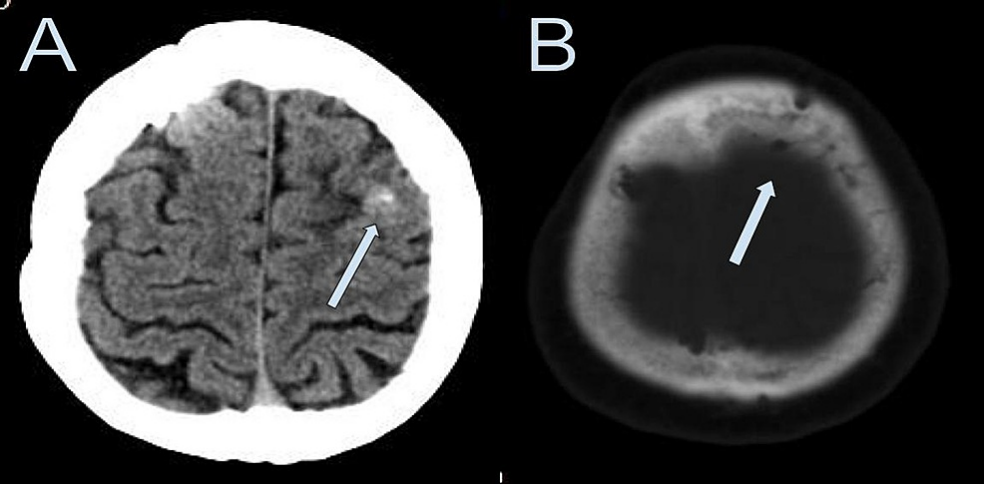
Axial head CT scan performed 15 years after the operation. (A) The small fragment of bone at the granuloma location is shown (white arrow). (B) The bone window image shows the bone defect at the internal and external table of the skull after the twist drill craniostomy (white arrow).
